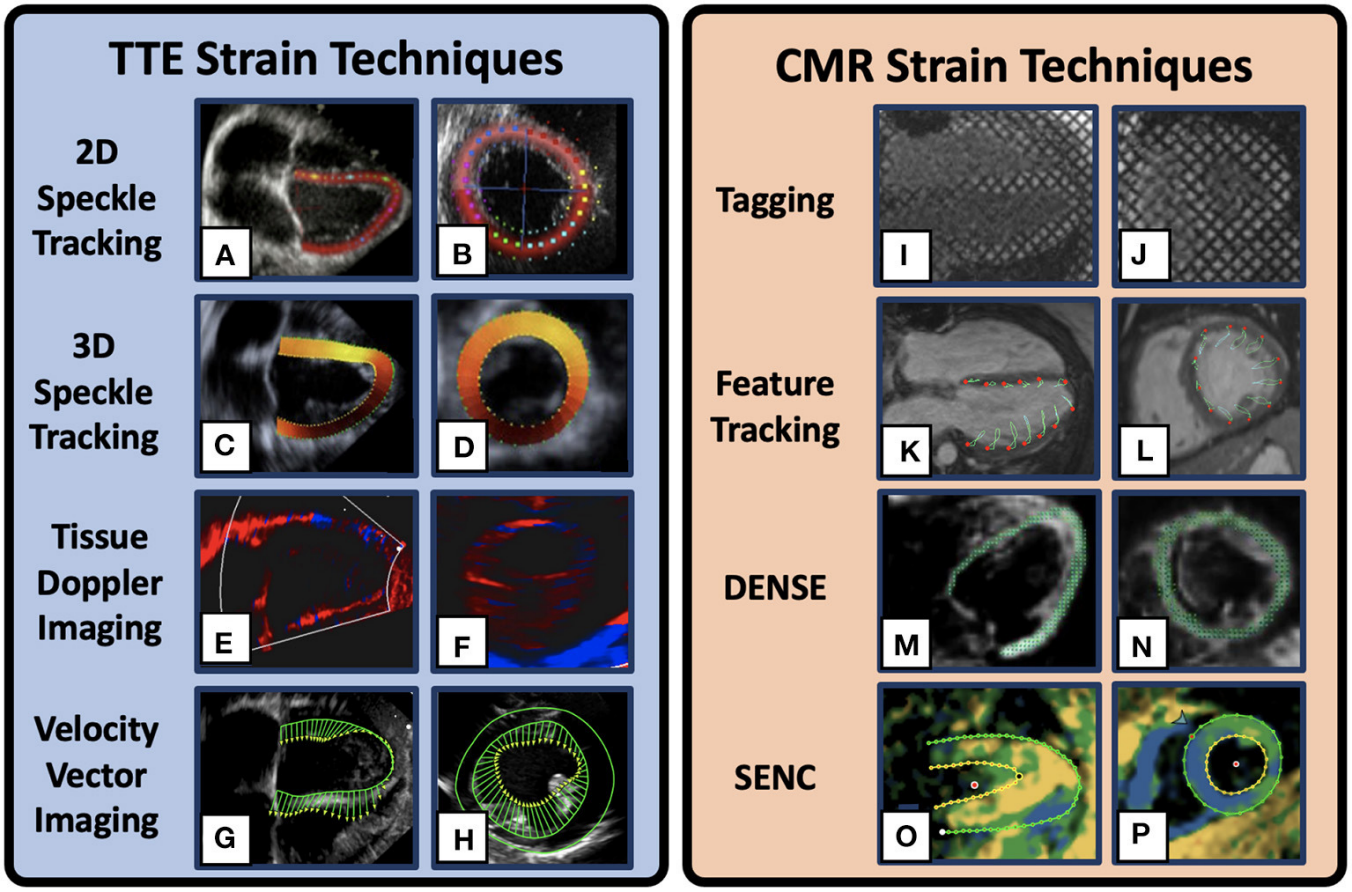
Example Cardiac Strain Imaging Techniques. 2D speckle tracking Transthoracic echocardiogram (TTE) image in the 4-chamber (A) and mid-ventricular short-axis (B)[adapted from Mele et al. (33)]. 3D speckle tracking TTE images in the 4-chamber (C) and short-axis (D)[adapted from Maffessanti et al. (34)]. Tissue Doppler imaging TTE images in the 2-chamber long-axis (E) and short axis (F) in a DMD patient. Velocity vector TTE images in the 4-chamber (G) and short axis (H) in a DMD patient. Cardiovascular magnetic resonance (CMR) strain method depicting myocardial tagging in the 4-chamber (I) and mid-ventricular short-axis (J) views in a DMD patient. CMR feature tracking example images in the 4-chamber (K) and short axis (L) views. Displacement Encoding with Stimulated Echoes (DENSE) example images in the 4-chamber (M) and short-axis (N) views [adapted from Cao et al. (35)]. Strain Encoded example images in the 4-chamber (O) and short-axis (P) view plane in a DMD patient. Multiple visualizations are available for each technique and the images presented here are only representative.
